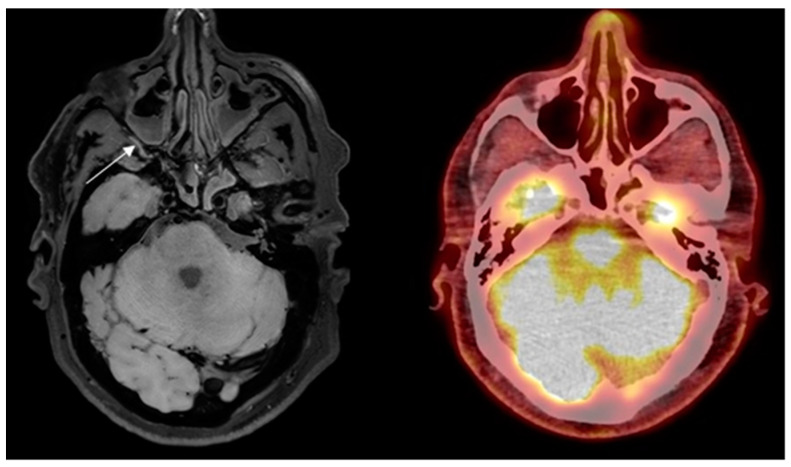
Control subject misinterpreted with GCA in the right maxillary artery on high-resolution T1-weighted 3D black blood without contrast enhancement Magnetic Resonance Imaging (arrow) and no signs of vasculitis on 2-deoxy-2-[^18^F]fluoro-D-glucose positron emission tomography/low- dose computed tomography.
